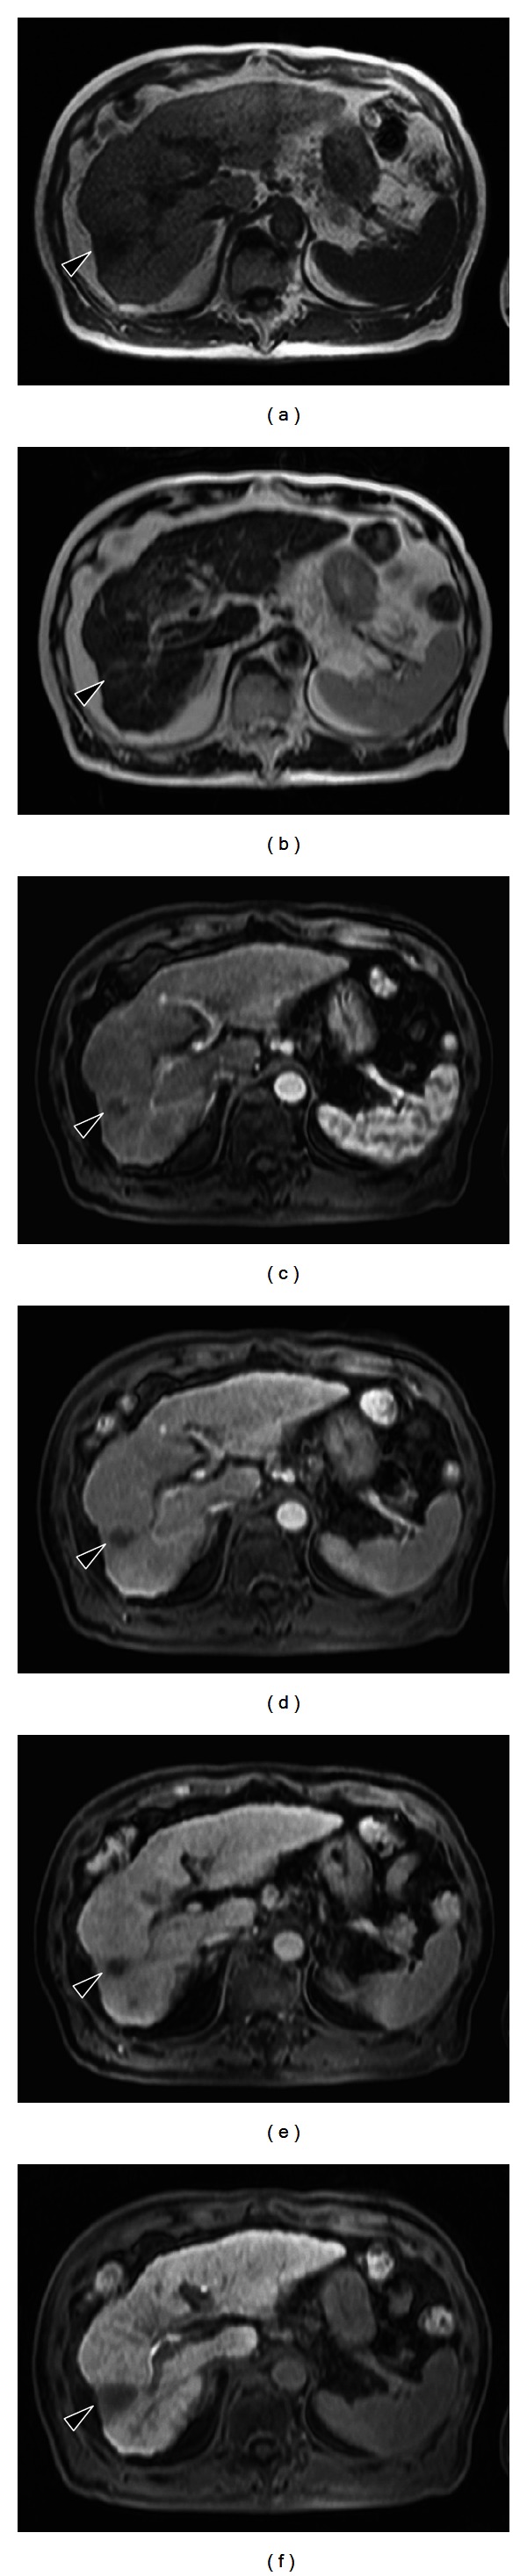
MR images obtained approximately four months after transcatheter arterial chemoembolization and radiofrequency ablation. (a) A hypodense area 1.5 cm in diameter is observed on T1-weighted image. (b) A hyperdense area is found on T2-weighted image. ((c)–(f)) Gadolinium ethoxybenzyl diethylenetriamine pentaacetic acid-enhanced images. In the arterial phase following contrast injection (c), the center of the tumor is enhanced intensely and homogeneously. In the delayed phase (d) and hepatobiliary phase (f), the tumor is completely washed out. e: portal phase. The tumor size has diminished compared to Figure 4.
